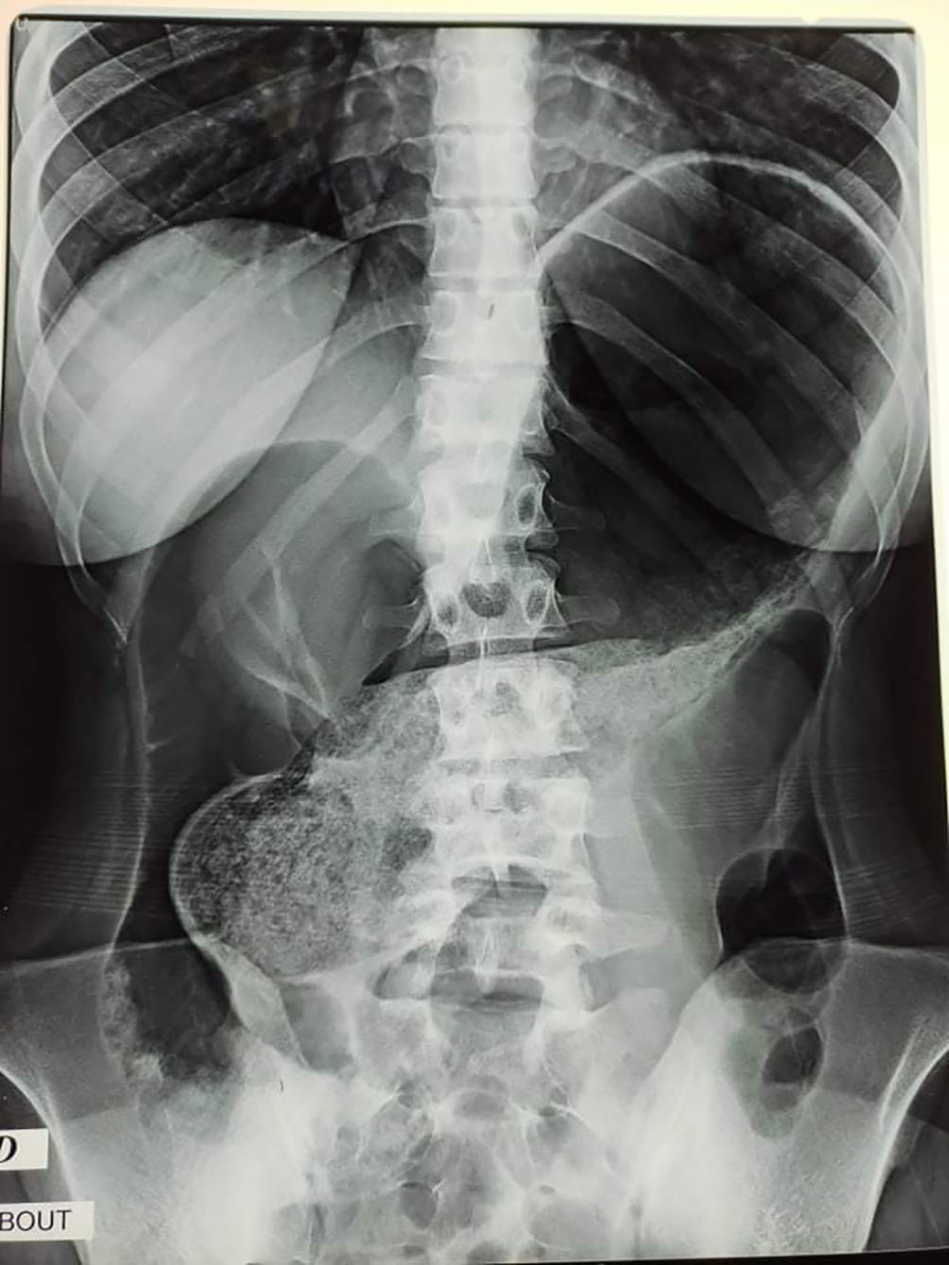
Abdominal X-ray showing the Significant distension of the colon reaching the epigastrium and the left hypochondrium.
